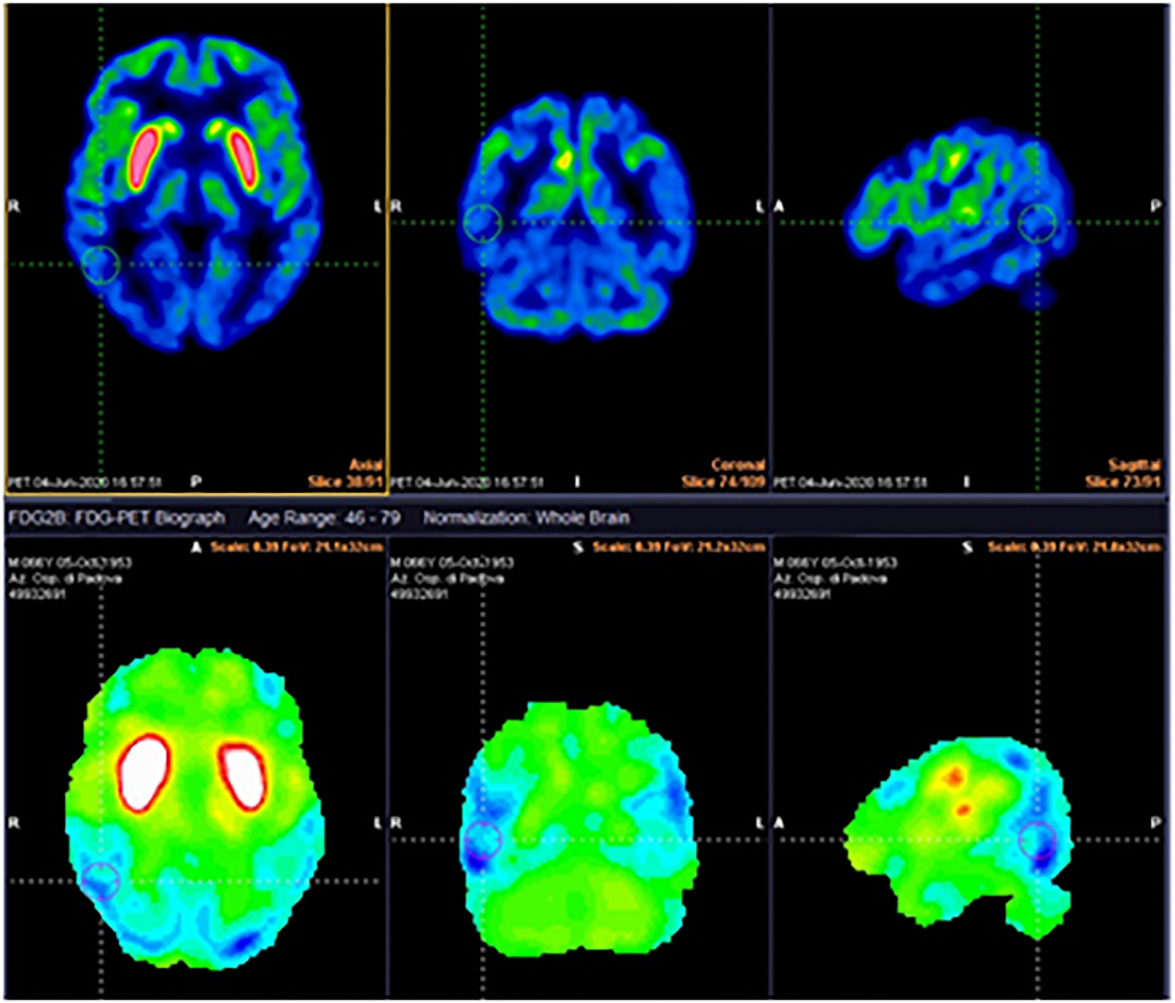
18‐FDG‐PET‐MRI showing marked hypermetabolism in the putamen bilaterally, especially compared to the caudate that appears less enhanced, and hypometabolism in the parieto‐temporal and occipital cortex bilaterally.
